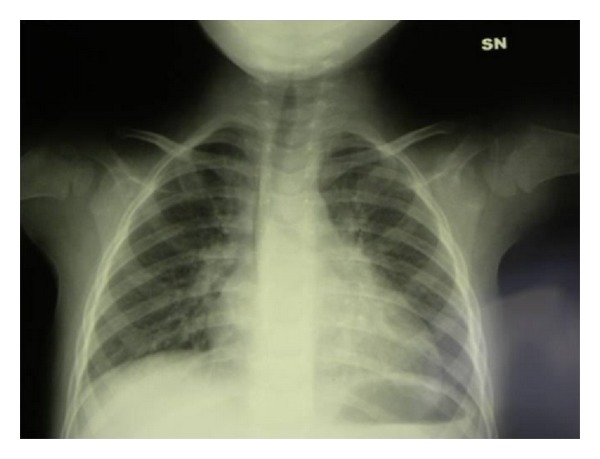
Chest X-ray: the chest X-ray showed reinforcement of the bronchial tree shadows, no bronchiectasis, and a markedly reduced thymic image.
